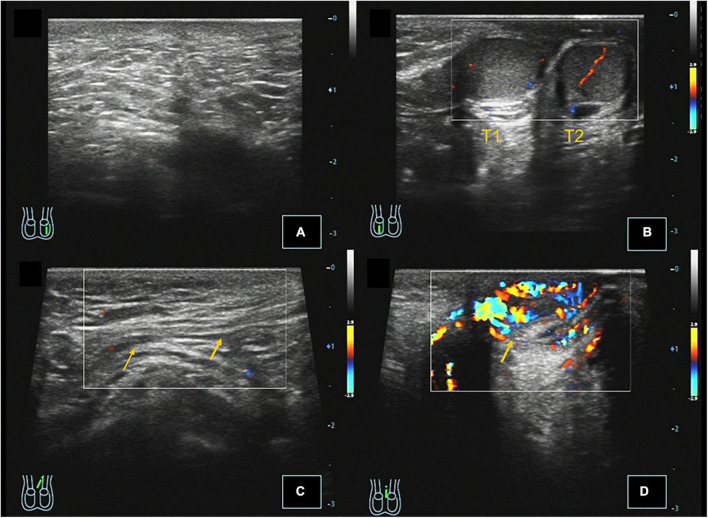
Case 3, 0.67 years, chief complaint: left scrotal emptiness. Preoperative ultrasound: (A) Longitudinal section of the left scrotum showing no testicular echo in the left scrotum. (B) Longitudinal section of the right scrotum showing that there were two testes echoes in the right scrotum, and CDFI showed that there were punctate blood flow signals in the testis parenchyma. (C) Longitudinal section of the left inguinal region showing that the left spermatic cord extends through the perineum to the right scrotum, and CDFI showed the punctate blood flow signal in the left spermatic cord. (D) Left scrotal longitudinal section showing hemiscrotal hemangioma, and CDFI showed that the blood flow signal is abundant in the mass.
